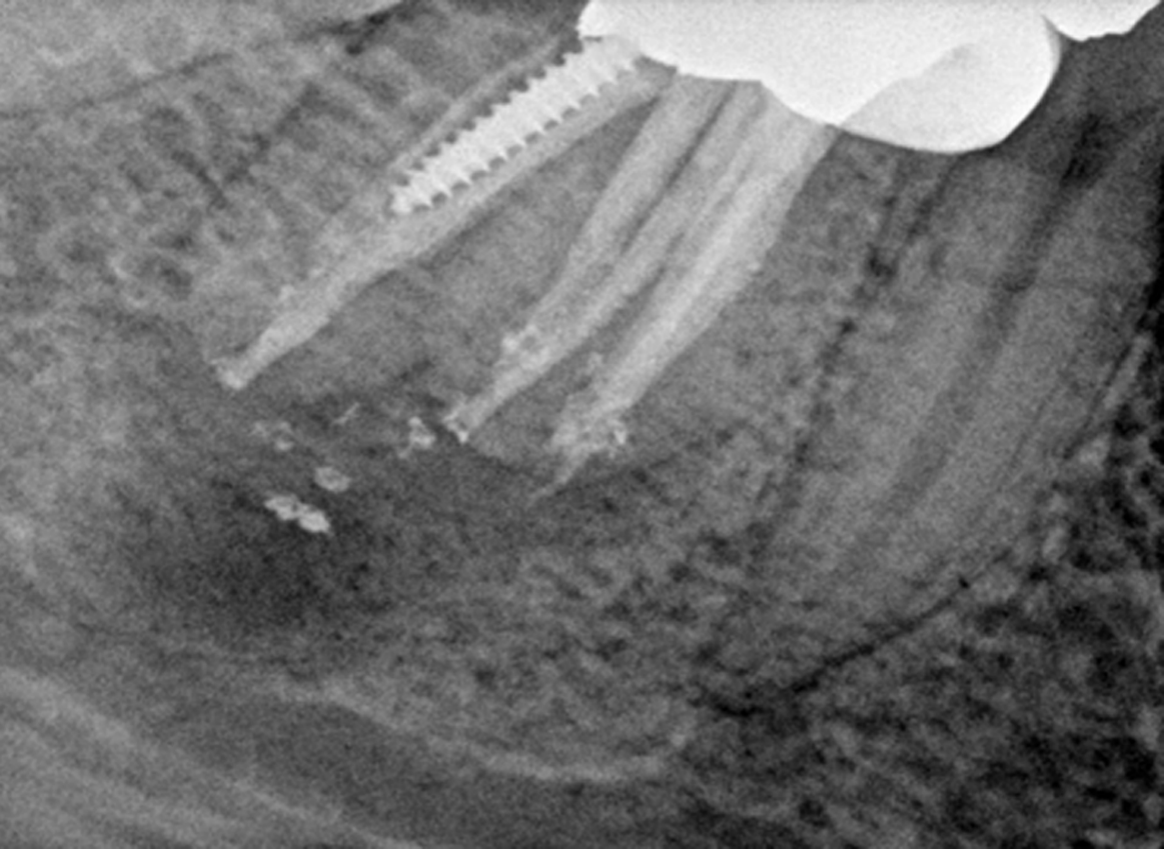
Three-month follow up radiograph
